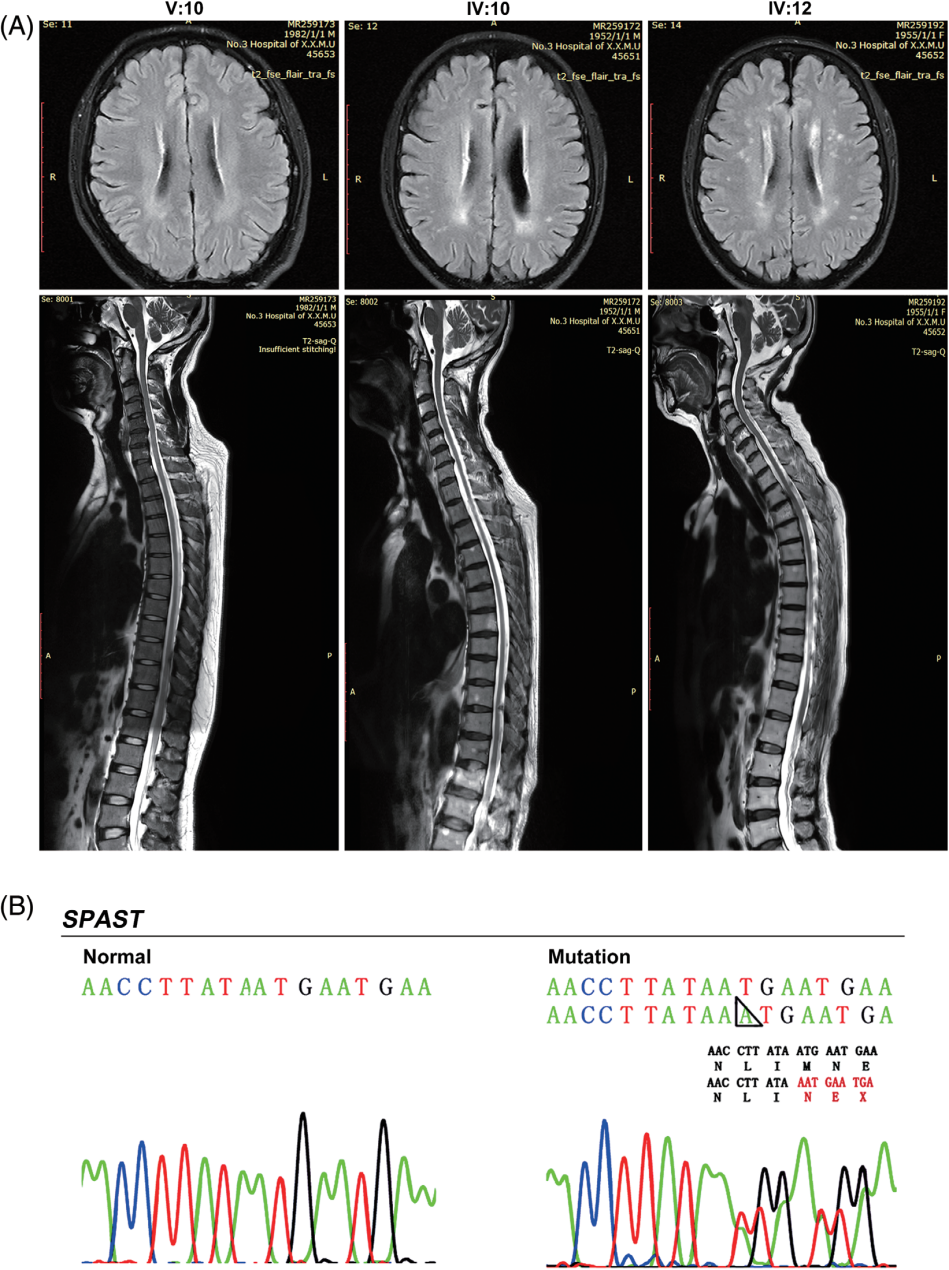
Magnetic resonance imaging (MRI) of the brain and spinal cord, and Sanger sequencing of the novel *SPAST* mutation. (A) T2‐weighted MRI of the brain (axial plane) and thoracic (sagittal plane) of patients V:10, IV:10, and IV:12 in Family 1. (B) The novel mutation was confirmed by Sanger sequencing. A black triangular box indicates the position of the *SPAST* mutations. The predicted amino acid sequence generated by the mutation is displayed below the wild‐type amino acid sequence. [Color figure can be viewed at wileyonlinelibrary.com]
